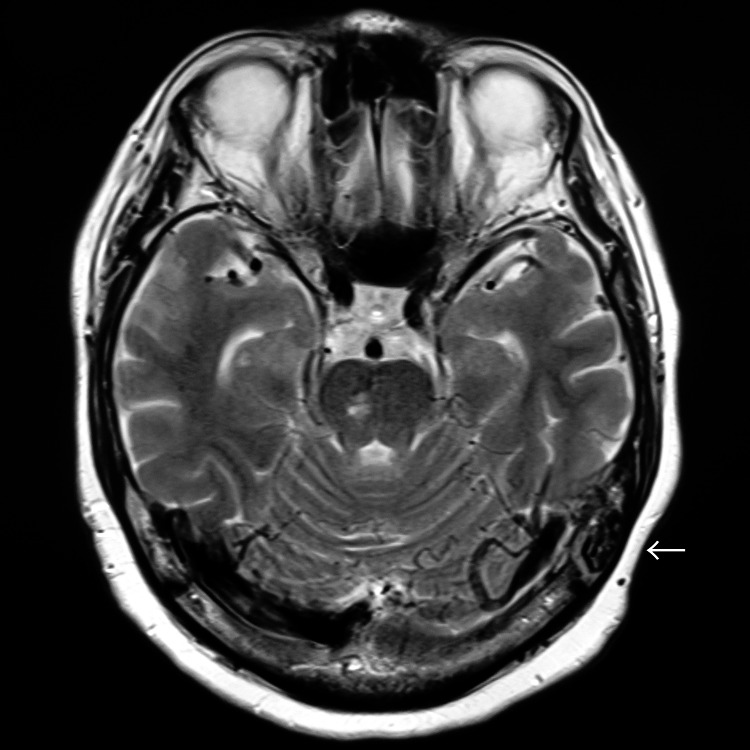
MRI T2-weighted image showing multiple dilated veins gathered around the left transverse sinus (white arrow) MRI: magnetic resonance imaging
